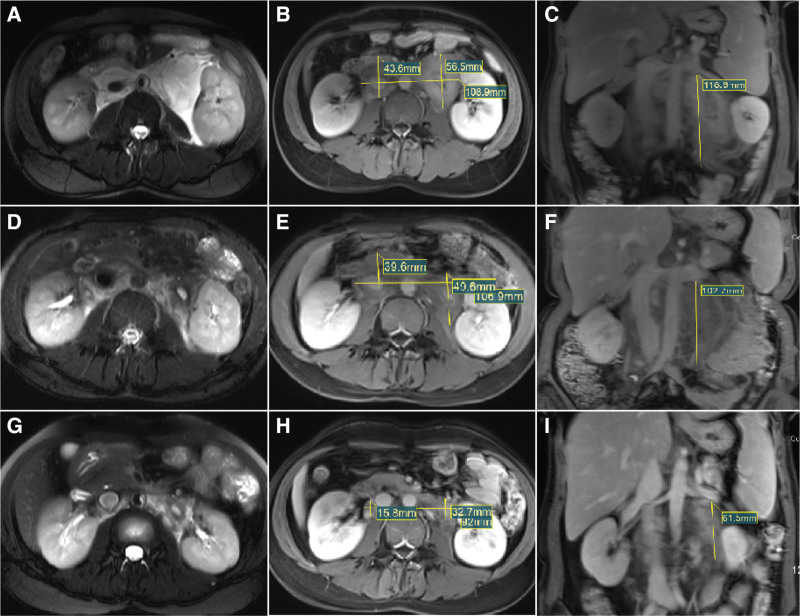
MRI images of a 32-year-old female patient before and after treatment. (A–C) MRI images from 1 year before admission. (A) Patchy hyperintensity in the retroperitoneal area on T2-weighted imaging. (B and C) Slight enhancement of the lesion and its extent on T1-weighted imaging. (D–F) MRI images after the first treatment. (D) Reduced T2 hyperintensity in the retroperitoneal patch, indicating improvement after treatment. (E and F) Significant reduction in the lesion area, reflecting a positive response to treatment. (G and I) Follow-up MRI images. (G) Decreased T2 signal intensity in the retroperitoneal area, indicating reduced inflammation. (H and I) Significant reduction in the lesion area, demonstrating the effectiveness of the treatment. Clinical Significance: MRI monitoring of retroperitoneal fibrosis shows a significant reduction in both the lesion area and T2 signal intensity after treatment, indicating a favorable treatment response and disease improvement. MRI = magnetic resonance imaging.
